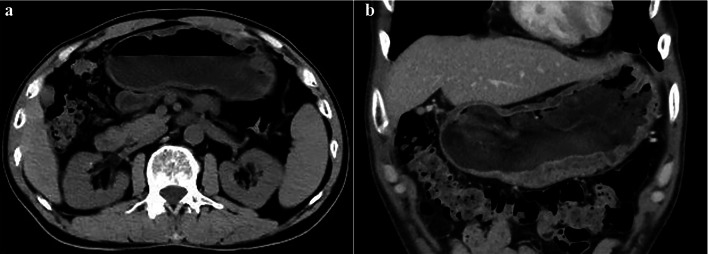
54-year-old man with GCP showing the diffuse thickening of gastric wall. a Axial unenhanced image reveals the thickened gastric mucosal folds pervading the large areas of gastric body. b Coronal venous phase CT shows multiple cysts in the submucosal layer
